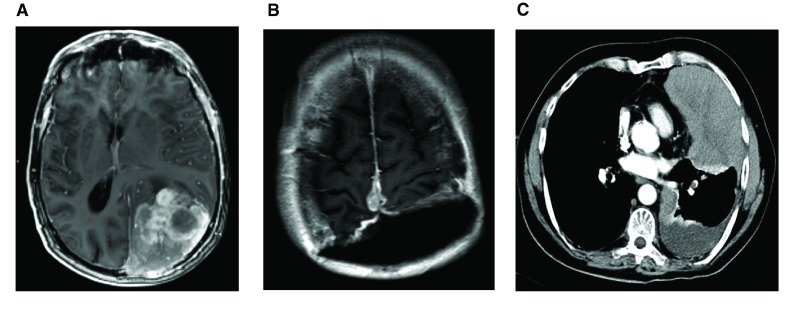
MRI and CT scanning of the original meninigioma intra-cerebral and at distance metastasis intra-pleural. A: T1 weighted image after administration of Gadolinium based contrast fluid shows a large extra-axial enhancing lesion in the left parieto-occipital region with local mass effect. Note that the sagittal sinus seems to be invaded.
